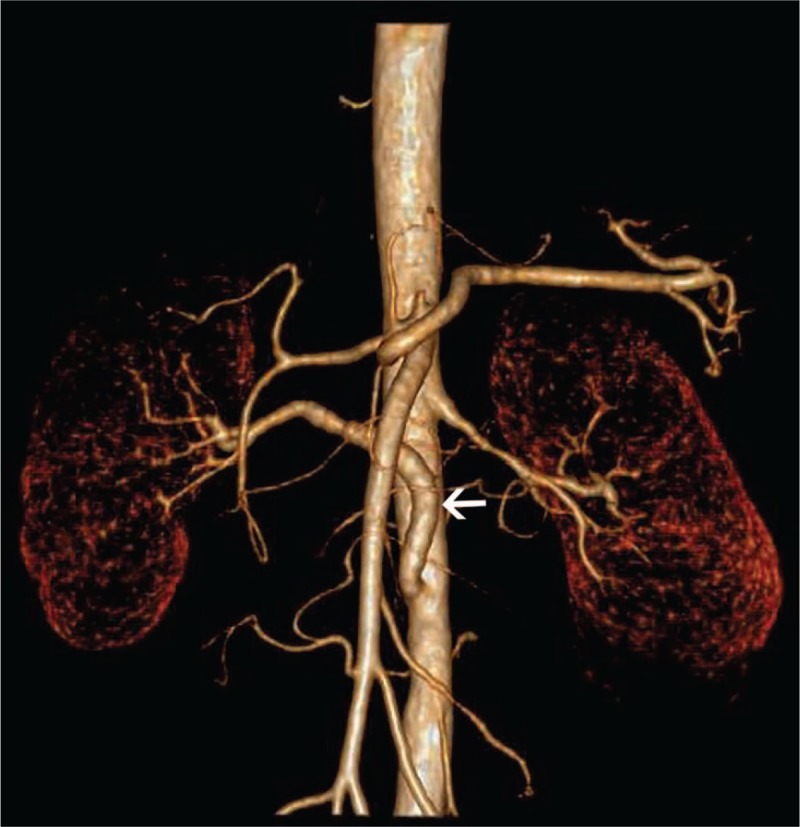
CTA: Four-year follow-up after reoperation revealed a normal PTFE graft (white) without restenosis or aneurysmal degeneration.
